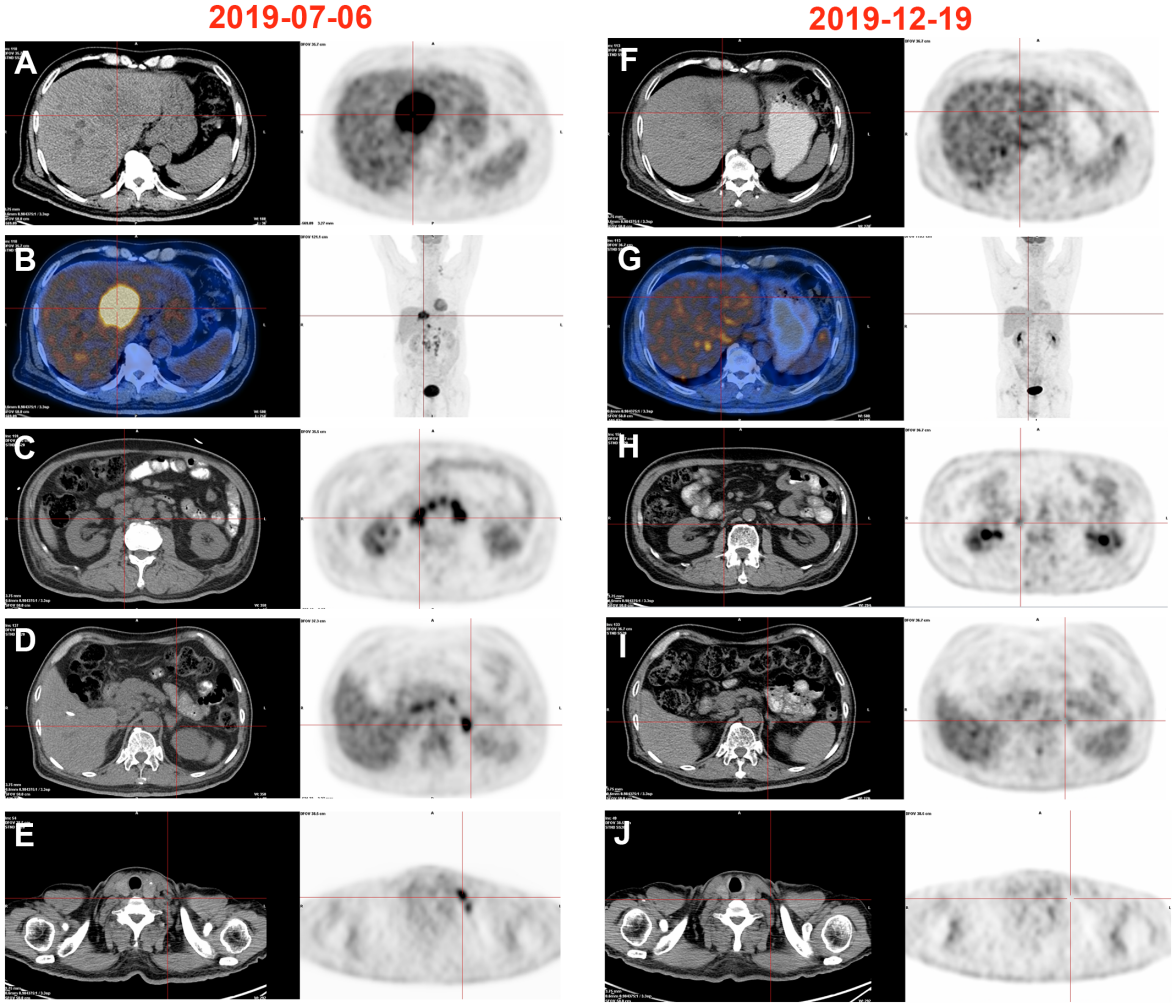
Changes of PET-CT images. (A–E) High metabolism in the left liver, retroperitoneum, left adrenal gland, and left supraclavicular lymphnode, 2019/07/06. (F–J) Hypermetabolism disappeared in the left liver, retroperitoneum, left adrenal gland, and left supraclavicular lymphnode, 2019/12/19.
